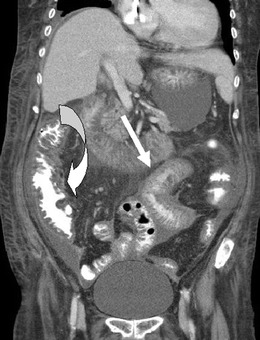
TB peritonitis in a CAPD patient presenting with non-specific abdominal symptoms and general malaise confirmed by microbiological evaluation of the dialysate seen on coronal CT with IV contrast demonstrating stranding of the mesentery, small (*straight arrow*) and large (*curved arrow*) bowel wall thickening features of which are non-specific and can also be seen with other causes of peritonitis
